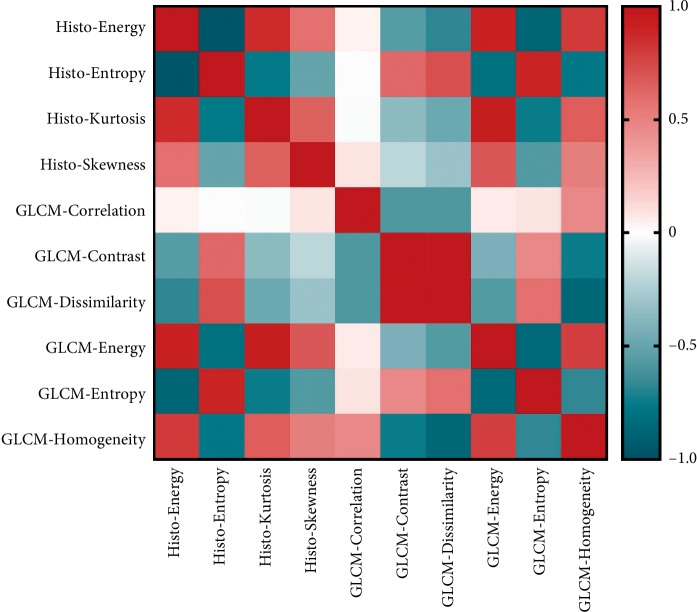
HOTMAP of MRI textural parameters.
